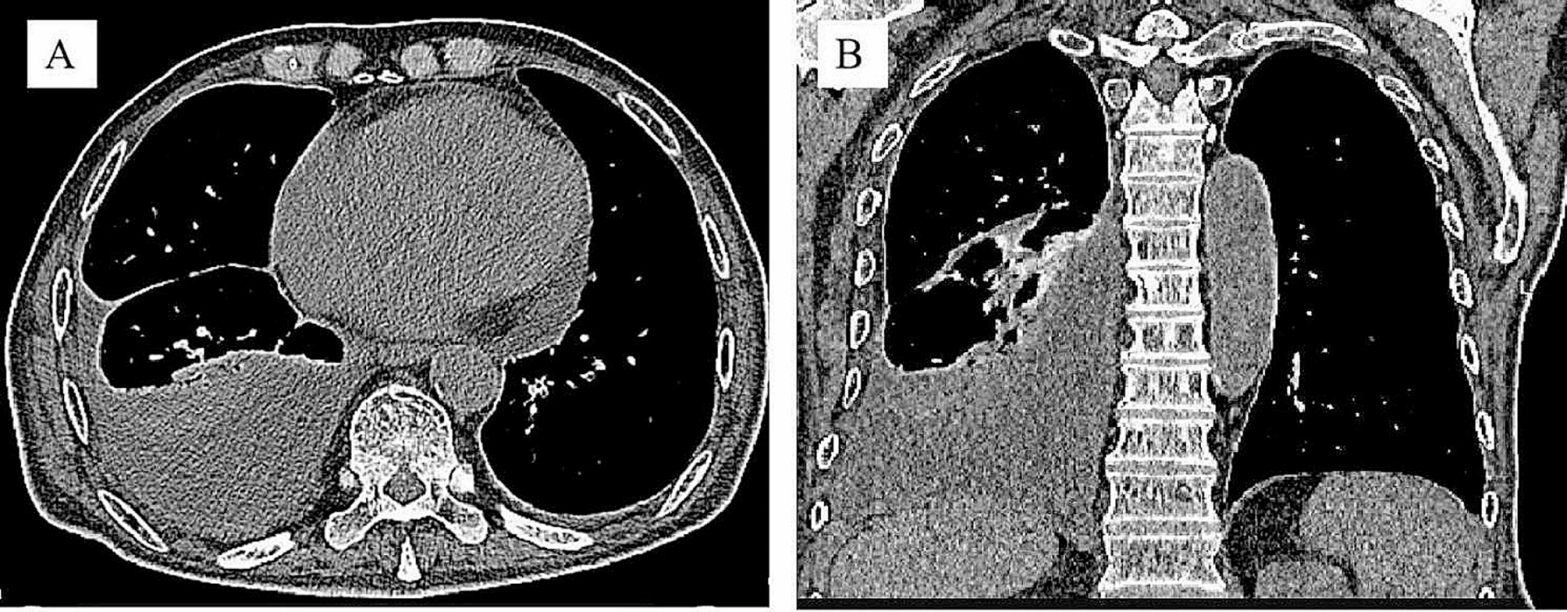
Radiological images of the chest CT. A, Axial scan showed a large right-sided pleural effusion. B, Coronal scan showed the pleural effusion was partially encapsulated
